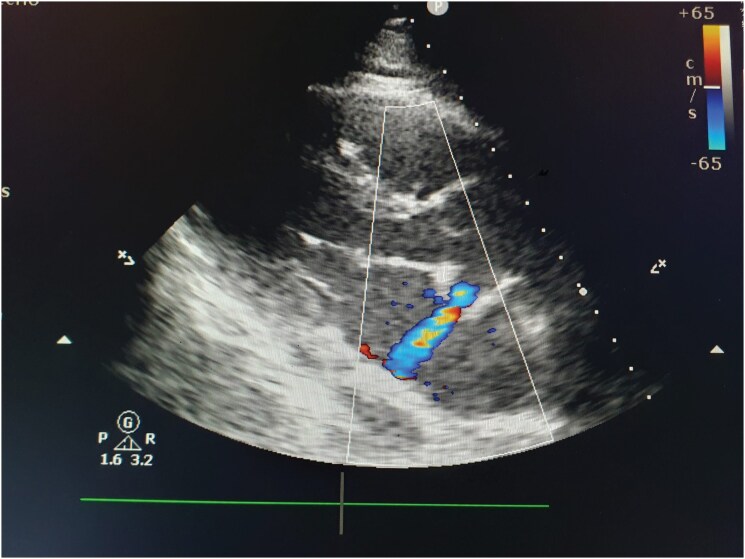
Colour-flow Doppler image demonstrating abnormal flow between the ascending aorta and the left atrium.
